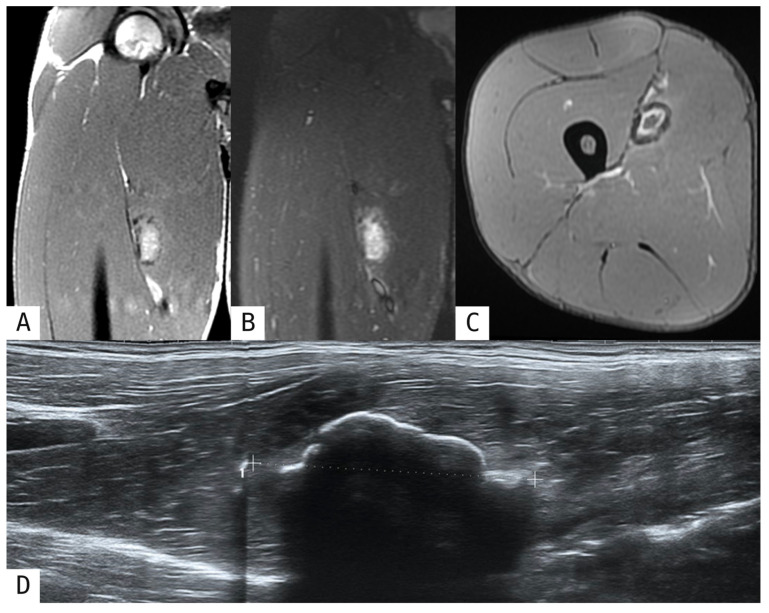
Post-traumatic myositis ossificans in the adductor longus. A 31-year-old soccer player presented with moderate pain in the right thigh two months after feeling a muscle strain while sprinting to get the ball. MRI coronal T1-weighted (A), coronal STIR (B), and axial MERGE (C) images showed a well-defined oval lesion with peripheral hypointensity and faint perilesional oedema in the adductor longus. Ultrasound examination was performed, with a longitudinal scan (D) confirming a mass with a peripheral calcified margin, consistent with the intermediate phase of myositis ossificans. The thin white dashed line indicates the maximum lesion diameter measured on ultrasound.
